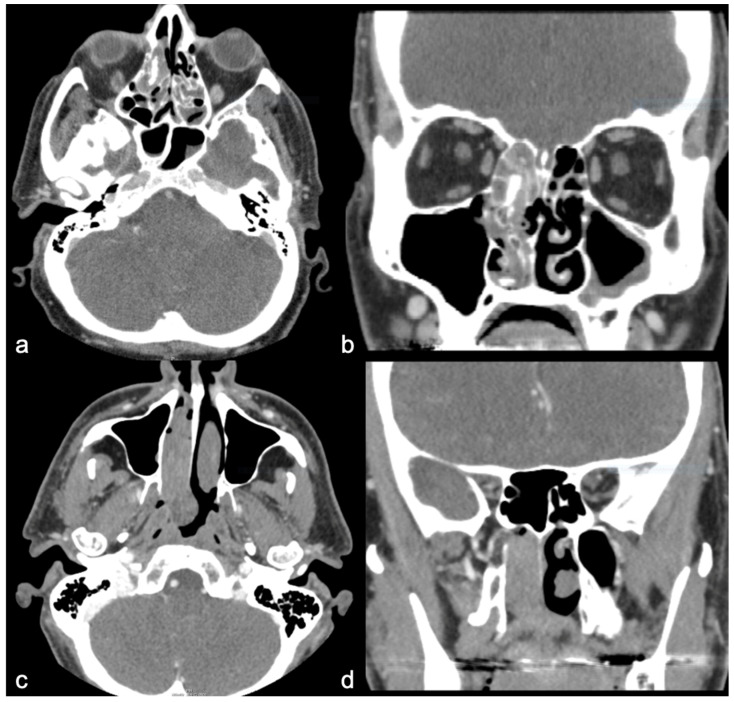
(a–d) Axial (left) and coronal (right) slices of two CT images of SNIP without SCC (case 1 (a,b) and case 2 (c,d)). Both tumors are unilateral (affecting only one side) without clear bone defects.
